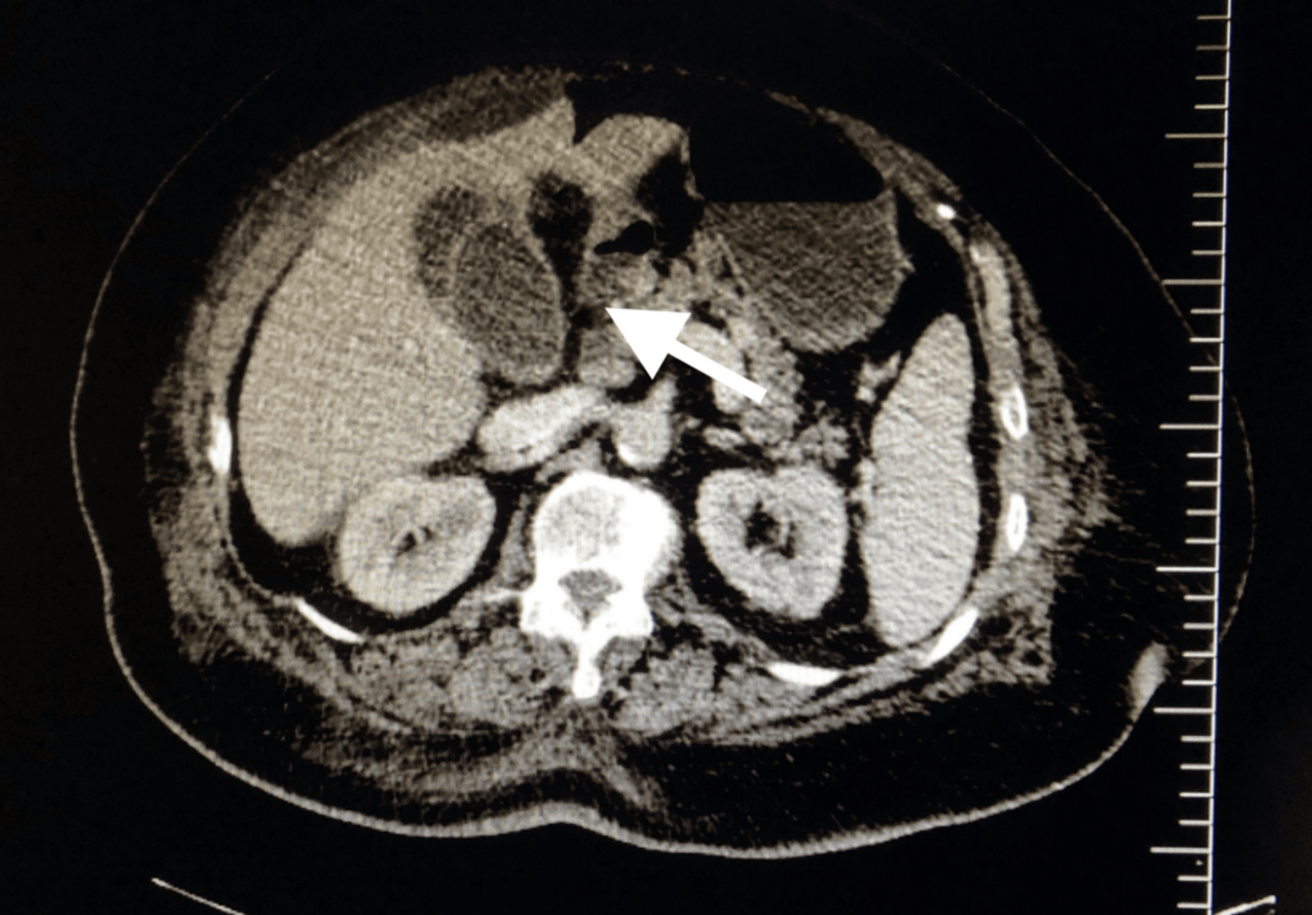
CT image of the patient; the arrow indicates a perforated gallbladder.
